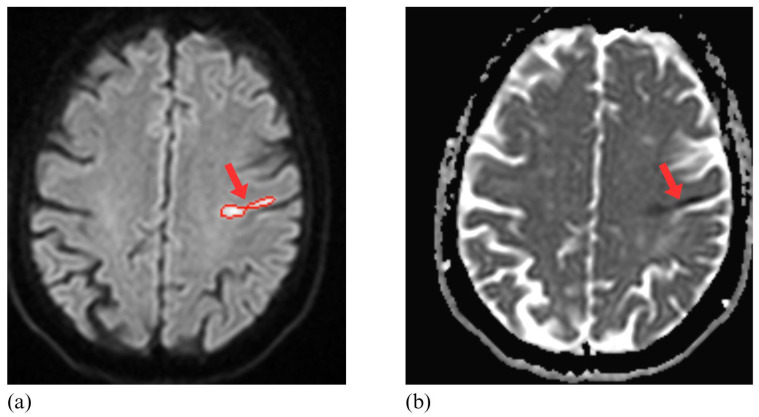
Detection of an Ischemic Lesion by Artificial Intelligence on DWI and ADC Maps. A 67-year-old woman presented with sudden-onset dysarthria and right upper limb weakness. The diffusion-weighted imaging (DWI) scan (a) and the apparent diffusion coefficient (ADC) map (b) correctly identified the ischemic lesion in the precentral gyrus (arrows) using the artificial intelligence (AI) software. On DWI (a), the AI delineation of the ischemic lesion is highlighted with red, indicating the automatically segmented area. The AI accurately delineated the ischemic area and generated an alert via the mobile application.
